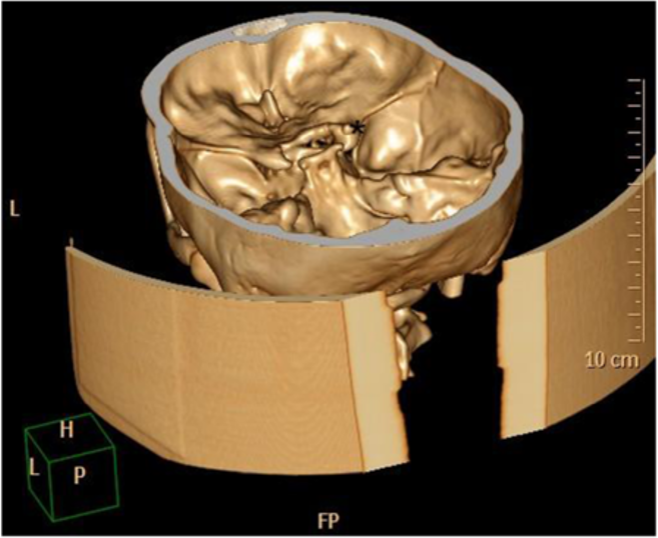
Robust processus clinoideus (*) on the right side – three-dimensional computed tomography reconstruction.
